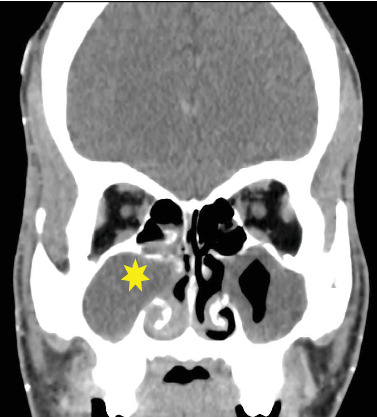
Coronal slice of postcontrast CT of the brain and sinuses viewed on soft tissue window, showing complete opacification (yellow star) of the right maxillary sinus, with widening of the osteomeatal complex.
